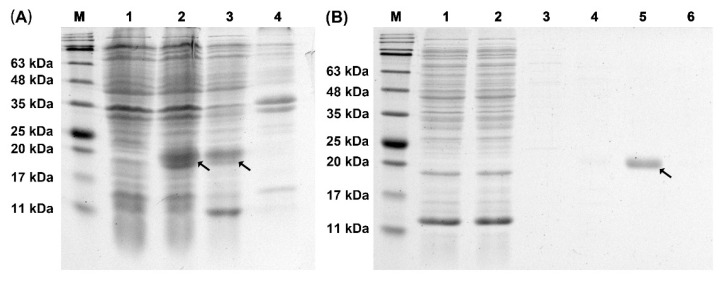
Expression and purification of recombinant protein TrufOBP4 analyzed by SDS-PAGE. (A) SDS-PAGE analysis of the expression of recombinant TrufOBP4. M: Protein molecular weight marker. 1: Protein sample without IPTG induction; 2: Protein sample with 0.5 mM IPTG induction. 3: The supernatant of protein sample with 0.5 mM IPTG induction after ultrasonic crushing. 4: The precipitation of protein sample with 0.5 mM IPTG induction after ultrasonic crushing. (B) SDS-PAGE analysis of the purification of recombinant TrufOBP4. M: Protein molecular weight marker. 1: Protein fluid after flowing through the column. 2–6: Protein samples were obtained with imidazole elution at different concentrations (0, 40, 100, 200 and 1000 mmol/L imidazole). The target protein was marked by an arrow.
